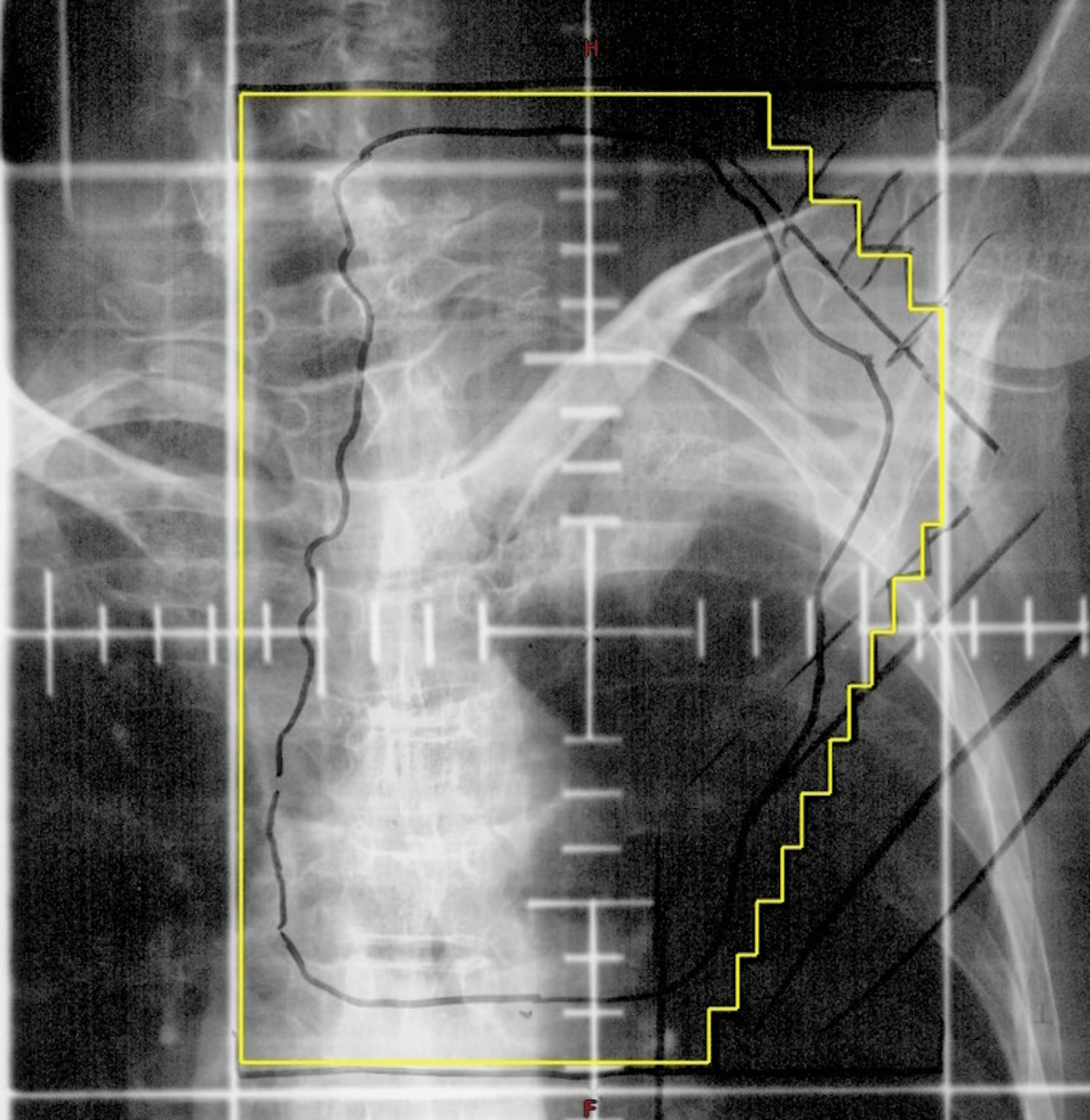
Chest X-ray showing the thoracic radiation plan targeting the left lung apex Posterior-anterior (PA) View
